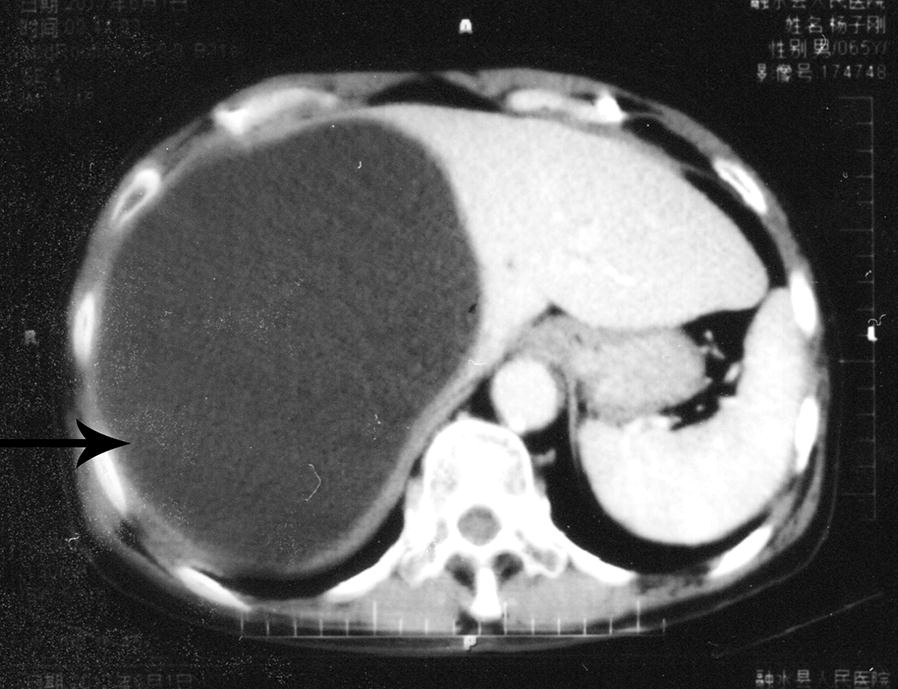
Abdominal ultrasonography detected a hydatid cyst (arrow) with a size of 17 × 12 × 20 cm (diameter) in the liver of the patient
